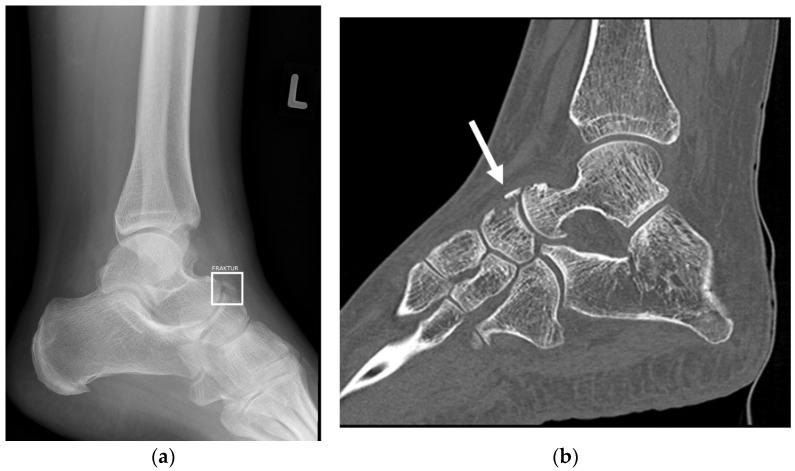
(a) shows a lateral radiograph of the left ankle. The bounding box (white) marks a fracture noted by the AI software. This was initially thought to be an osteophyte by both the resident and the board-certified radiologist. (b) Sagittal CT imaging confirms a true positive fracture of the Navicular (white arrow).
